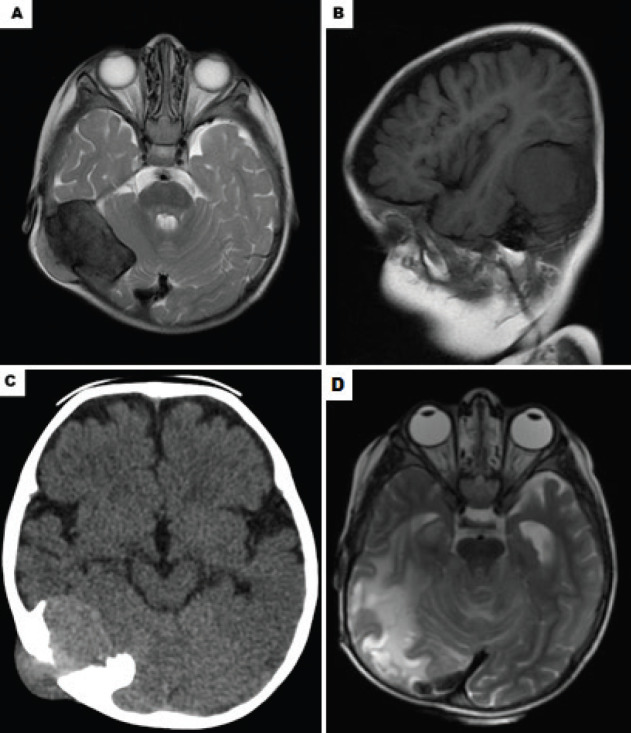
Imaging exams highlighting the injury of the patient. (A): Axial contrast-enhanced CT scan showing neoplastic lesion with an epicentre on the skullcap of the right temporo-occipital transition with intracranial and extracranial expansion. (B): Sagittal contrast-enhanced CT scan showing the same lesion. (C): Magnetic resonance imaging shows a highly enhancing tumour with epicentre in the right side of the posterior cranial fossa. (D): Computerizsd tomography 3 years after the last surgery, the patient accompanied only with chemotherapy and radiotherapy.
